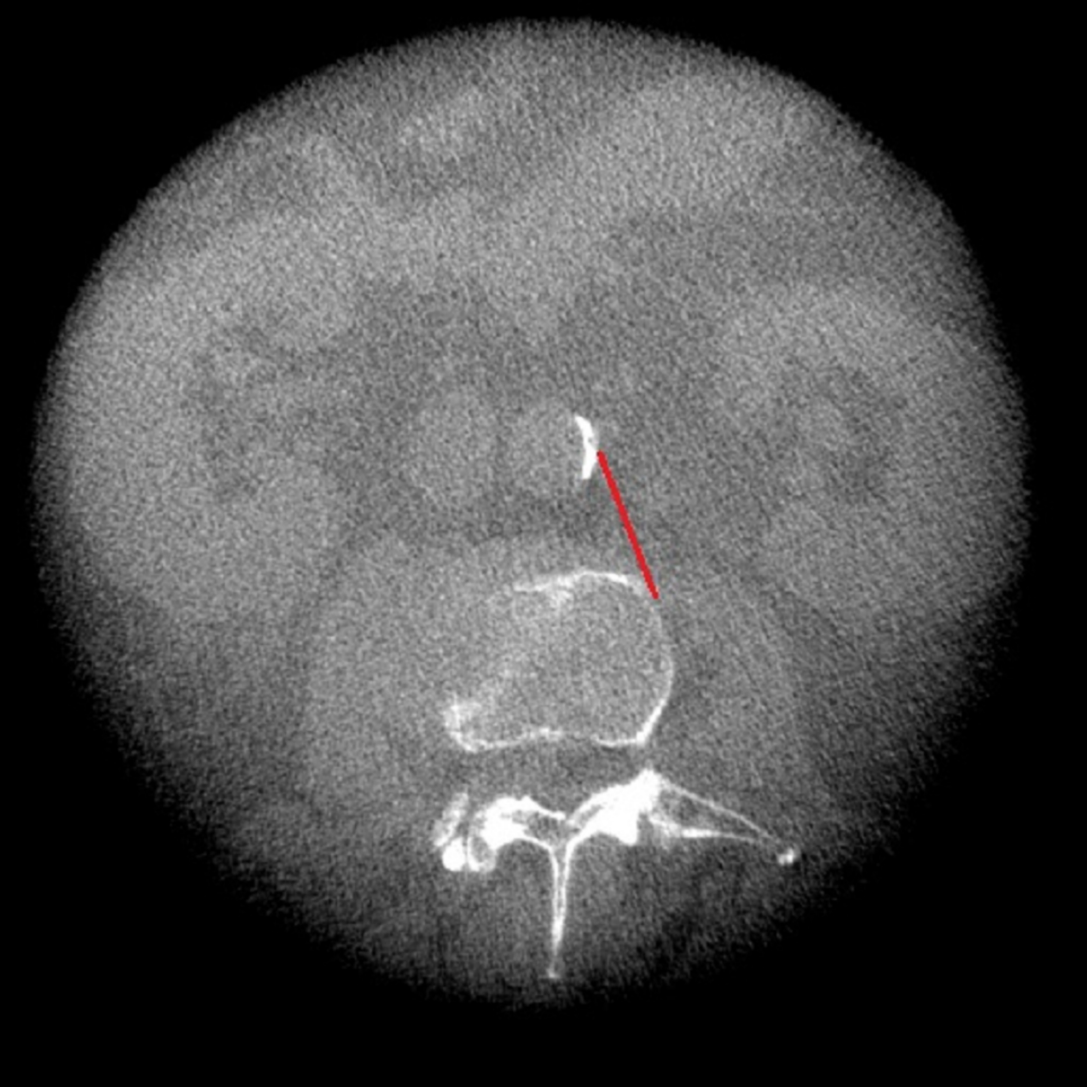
Intraoperative corridor measurement using CT of the same patient as in figure 1 at L2-L3 disc space as indicated by the red line CT: computed tomography
